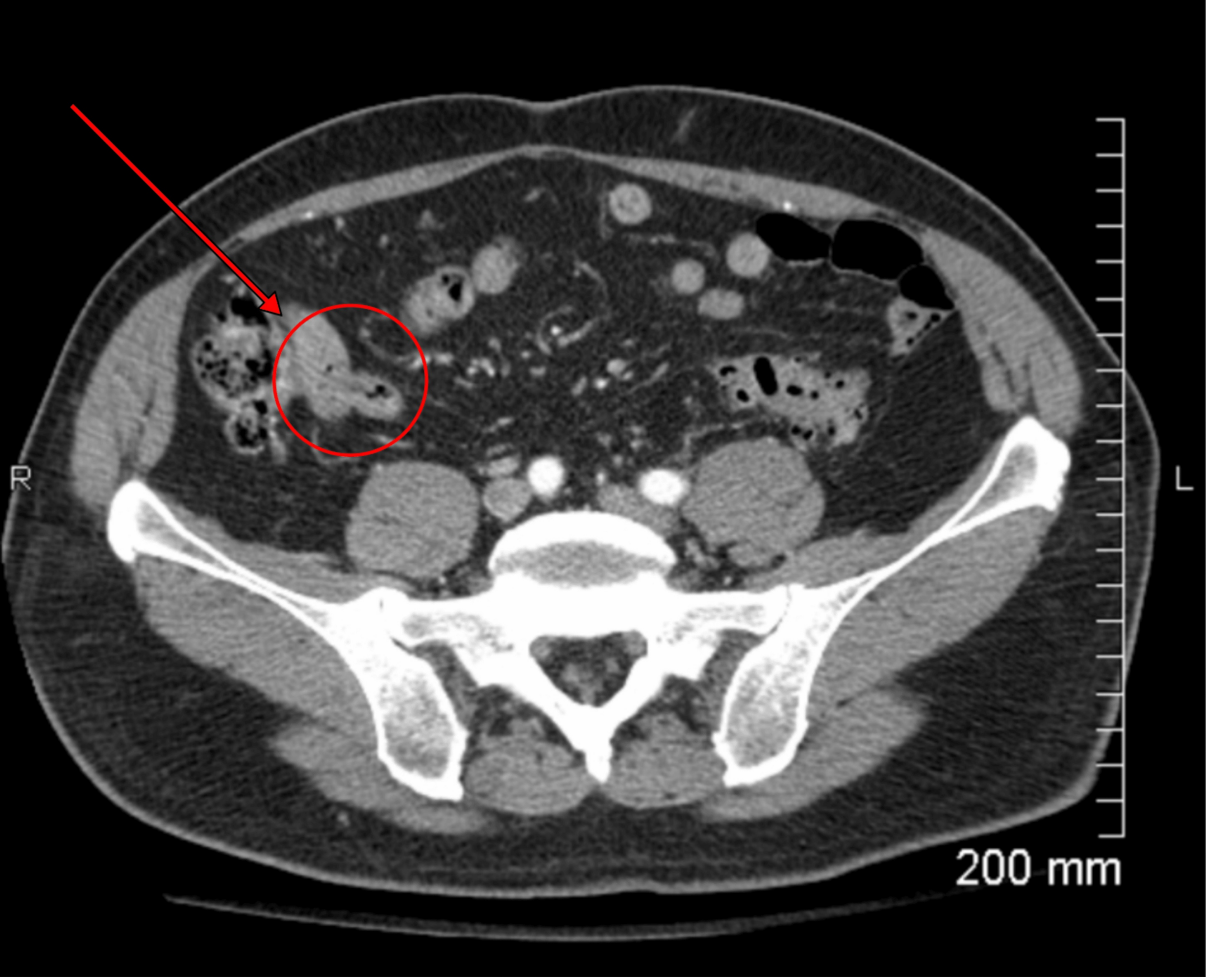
Contrast-enhanced CT of the abdomen and pelvis. Contrast-enhanced CT of the abdomen and pelvis demonstrating an elongated, retrocecal appendix measuring approximately 9.1 mm in diameter, containing an obstructing appendicolith with surrounding inflammatory changes, findings consistent with acute appendicitis. The area of interest is denoted by the red arrow.
